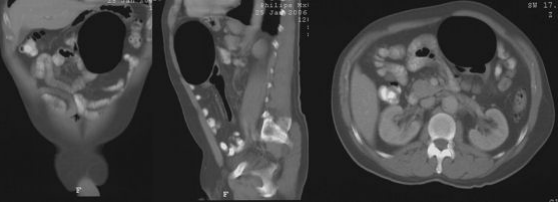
Selected coronal, sagittal and axial sections from CT scan demonstrating a large gas filled structure that appeared to be arising from the top of the sigmoid colon.
